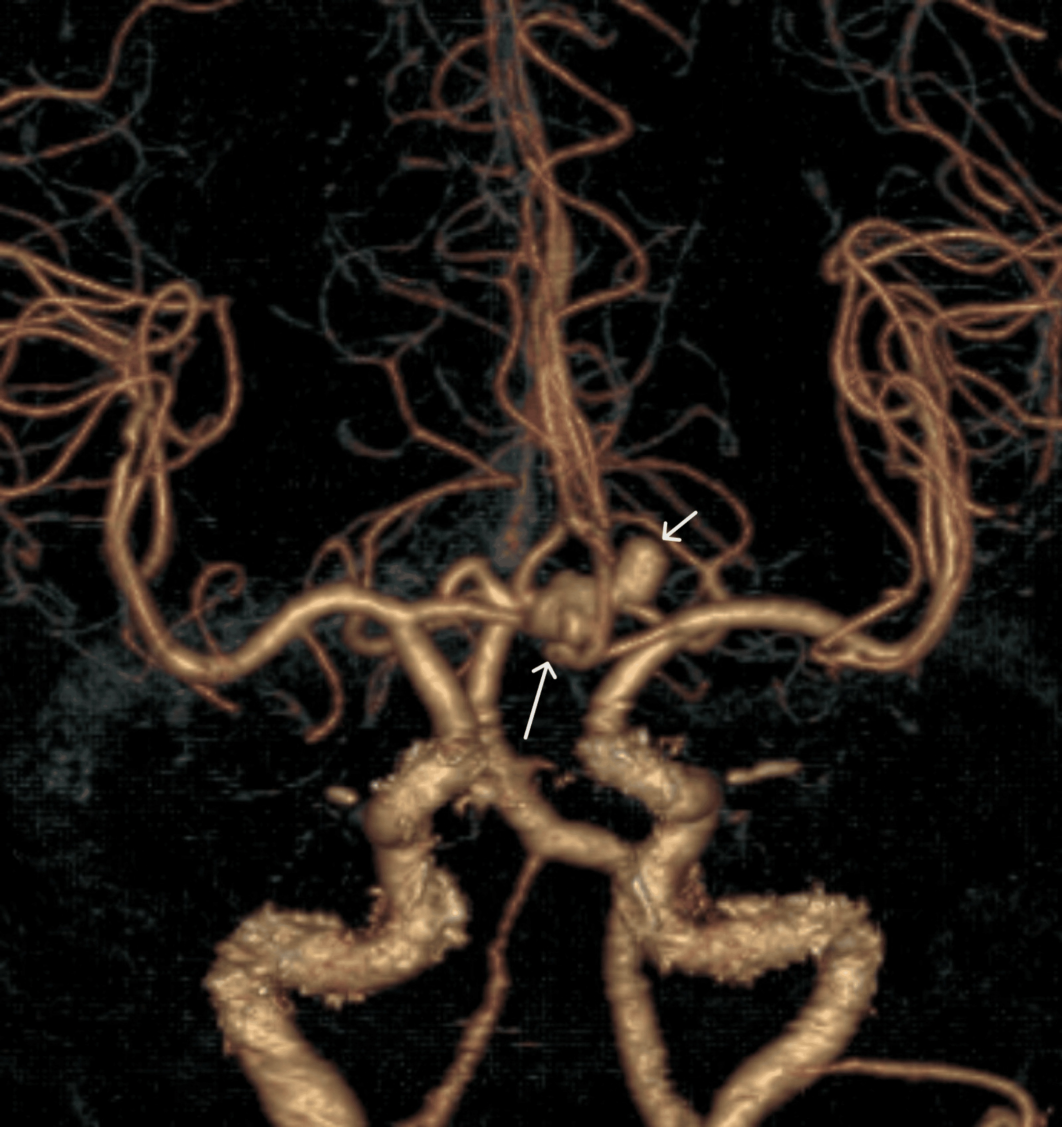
Axial CTA volume-rendered image demonstrating two aneurysms: one involving the ACOM (long white arrow) measuring 9.3 × 7.8 mm and the other involving the left ACA (short white arrow) measuring 5.8 × 5.5 mm CTA: computed tomography angiography, ACOM: anterior communicating artery, ACA: anterior cerebral artery
